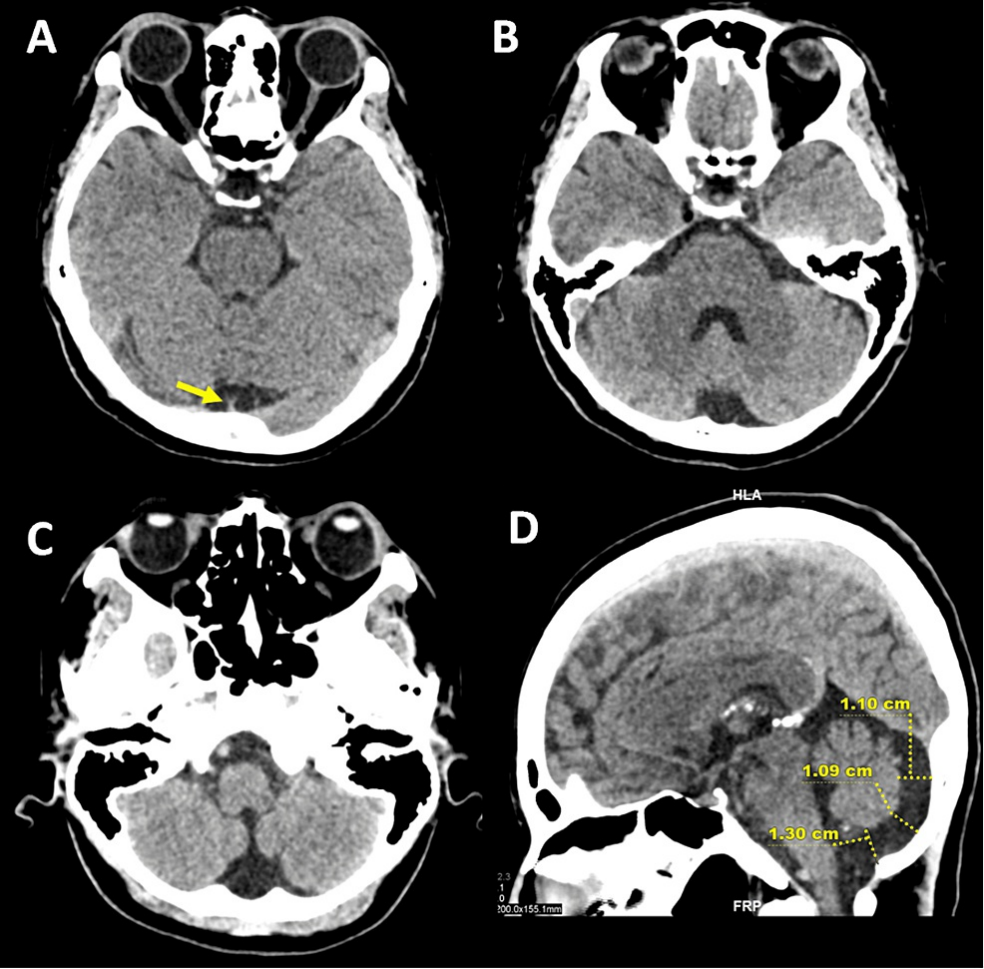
(A, B, C) A non-contrast CT brain in the axial plane reveals an enlarged retrocerebellar CSF space with normal vermis, cerebellar hemispheres, and a fourth ventricle. There was no hydrocephalus or mass effect on the cerebellum. Falx cerebelli is undisplaced (arrow). (D) The midsagittal plane demonstrates the diameter of the cisterna magna; the maximum measurement was obtained from the posterior lip of the foramen magnum to the caudal margin of the inferior vermis. There is no upward sloping of the tentorium cerebelli.
